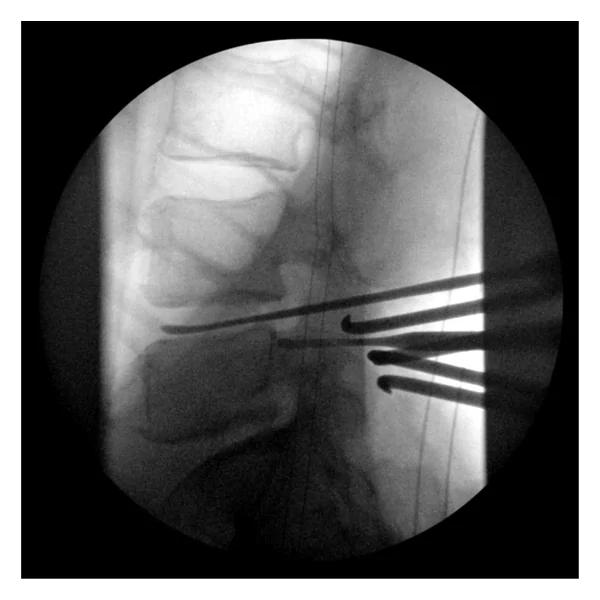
Intraoperative lumbar radiographs demonstrate debridement of the disc space between L4 and L5.
